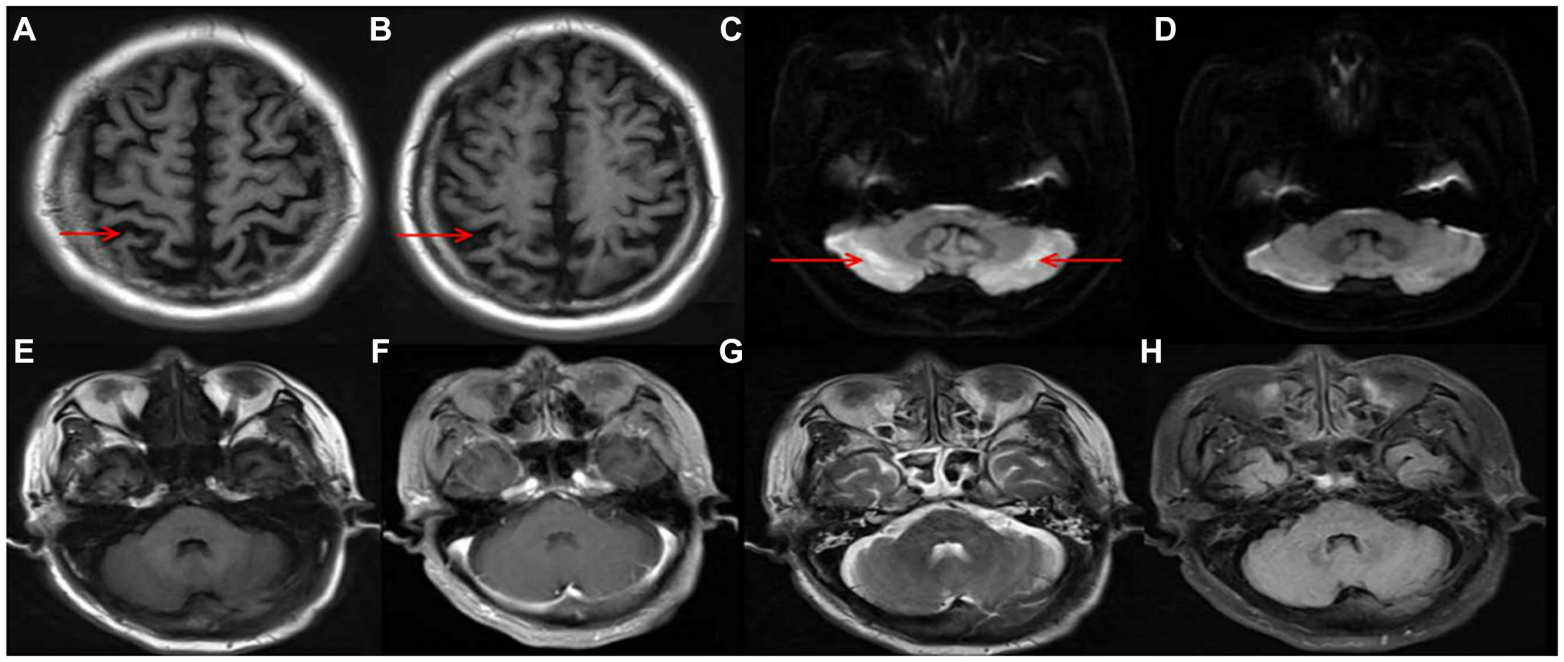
Cortical atrophy in T1 (A,B); symmetric hyperintensity in the bilateral lateral cerebellar hemispheres, predominantly manifested in DWI (C); reversal of the of cerebellar lesions after treatment (D); T1/ enhancement/ T2/ FLAIR image no significant changes in cerebellar (E–H).
